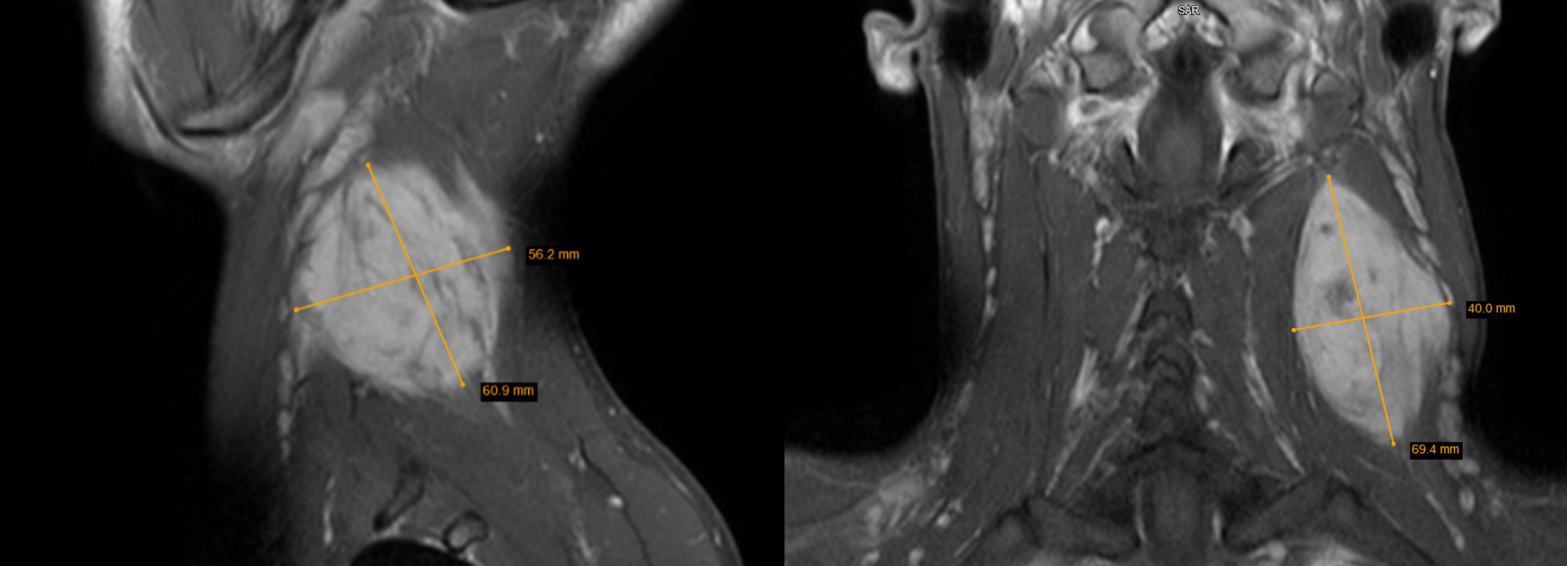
Magnetic resonance imaging (left—sagittal view; right—coronal view) of a mass in the paraspinal compartment of the perivertebral space measuring 69 × 40 × 56 mm.
